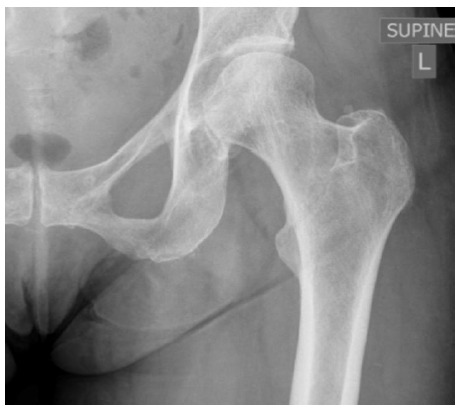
Case 4: X-ray of the left hip one week after the injury
